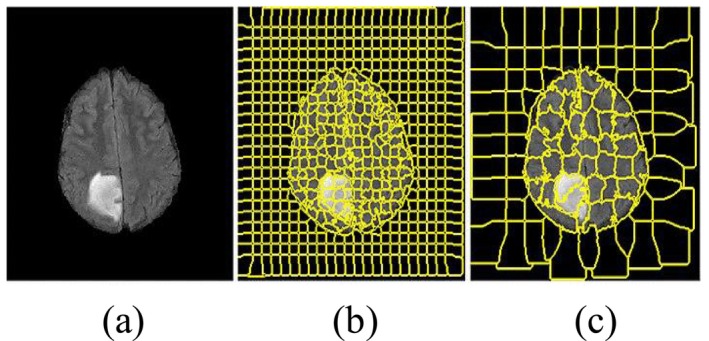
The superpixel‐based segmentation of brain tumor. (a) The input FLAIR MRI image of the grade II tumor, (b) the superpixel segmentation of the MRI image with different thresholds (m = 0.2, S = 10, and grid size 10 × 10), and (c) the superpixel segmentation at S = 20, m = 0.3 and grid size 20 × 20 [28].
